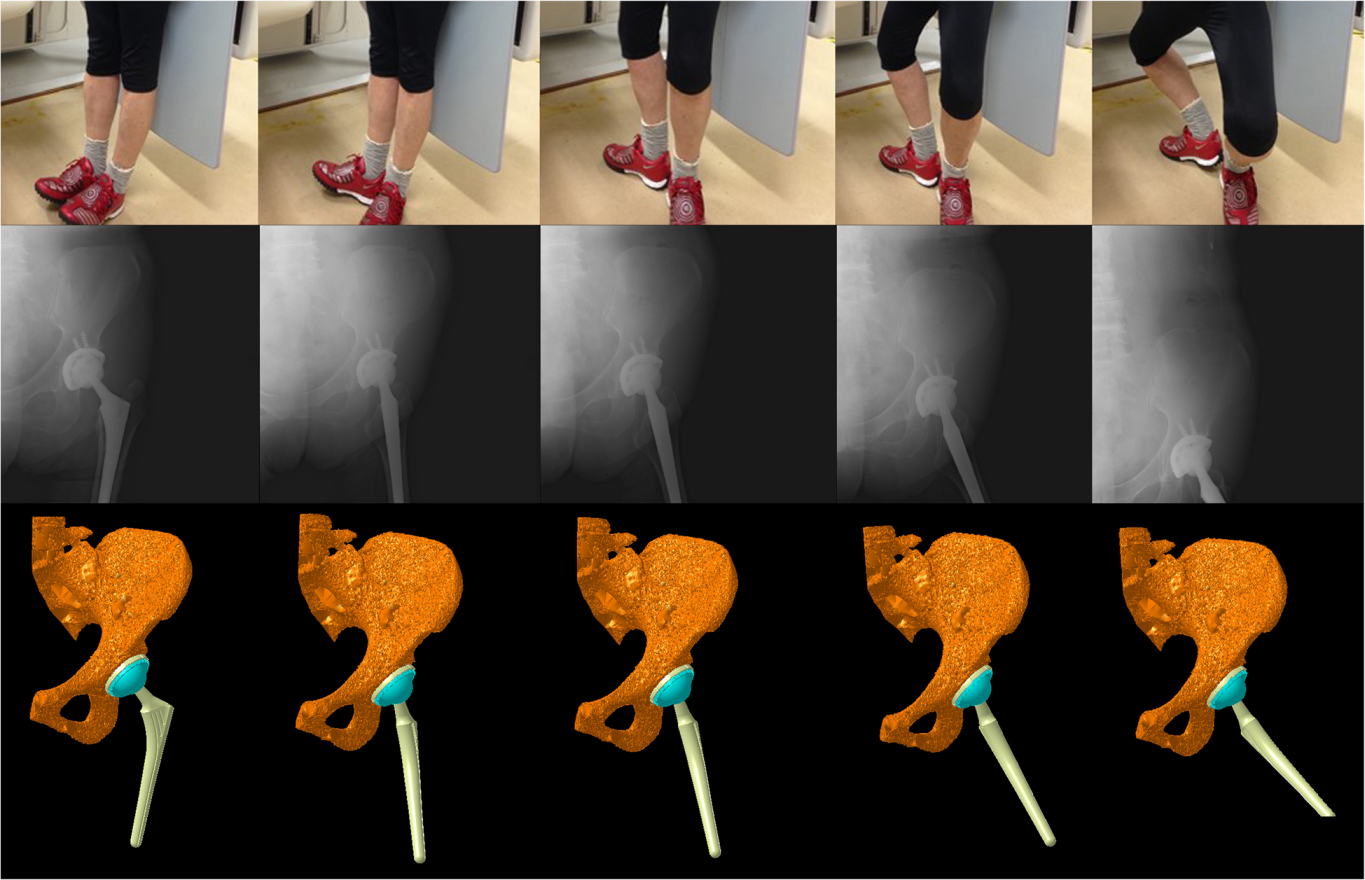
The hip motions during the ballet movement plié were captured as continuous X-ray images using a flat panel X-ray detector (*middle stand*) to reconstruct three-dimensional images of the replaced hip joint (*lower stand*) using image-matching techniques
